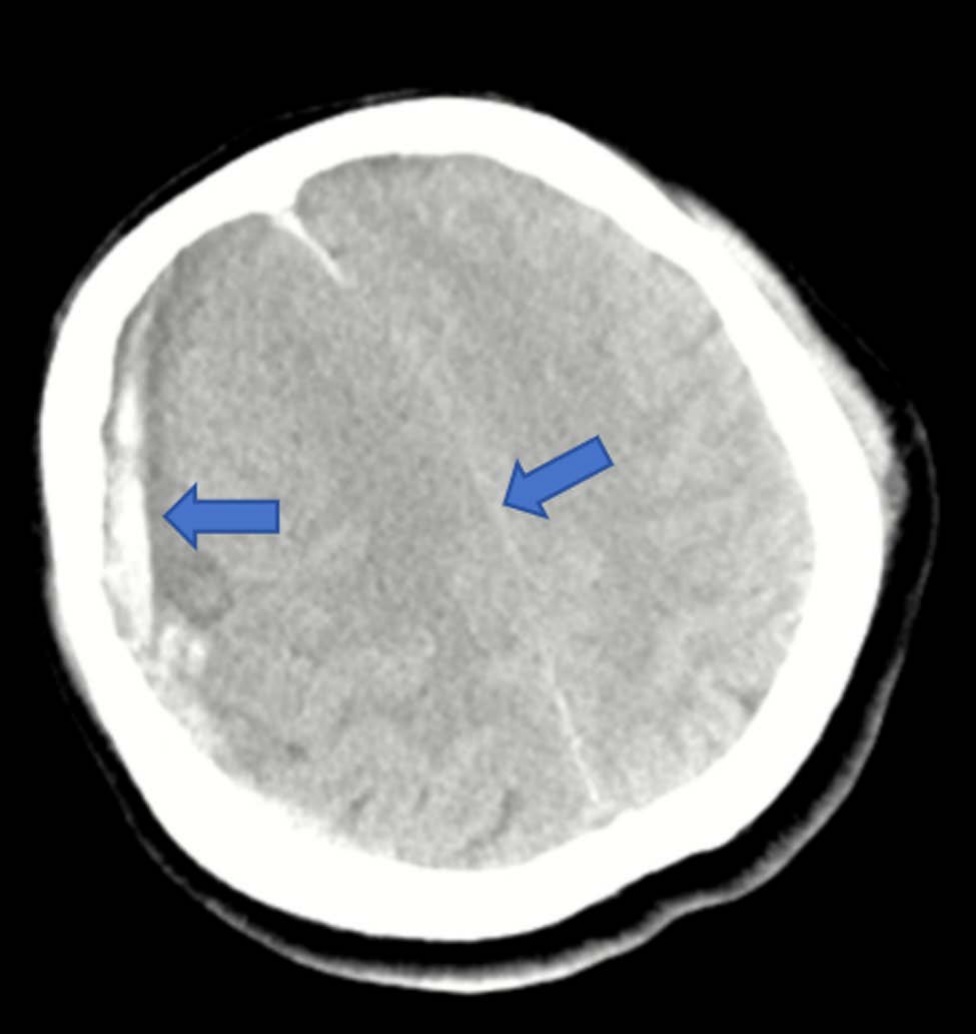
Cranial CT findings. Non-contrast cranial CT demonstrating a right subdural hematoma (arrow) producing a 10-mm midline shift. Bilateral pulmonary contusions are also visible on the same scan series. CT = computed tomography.
